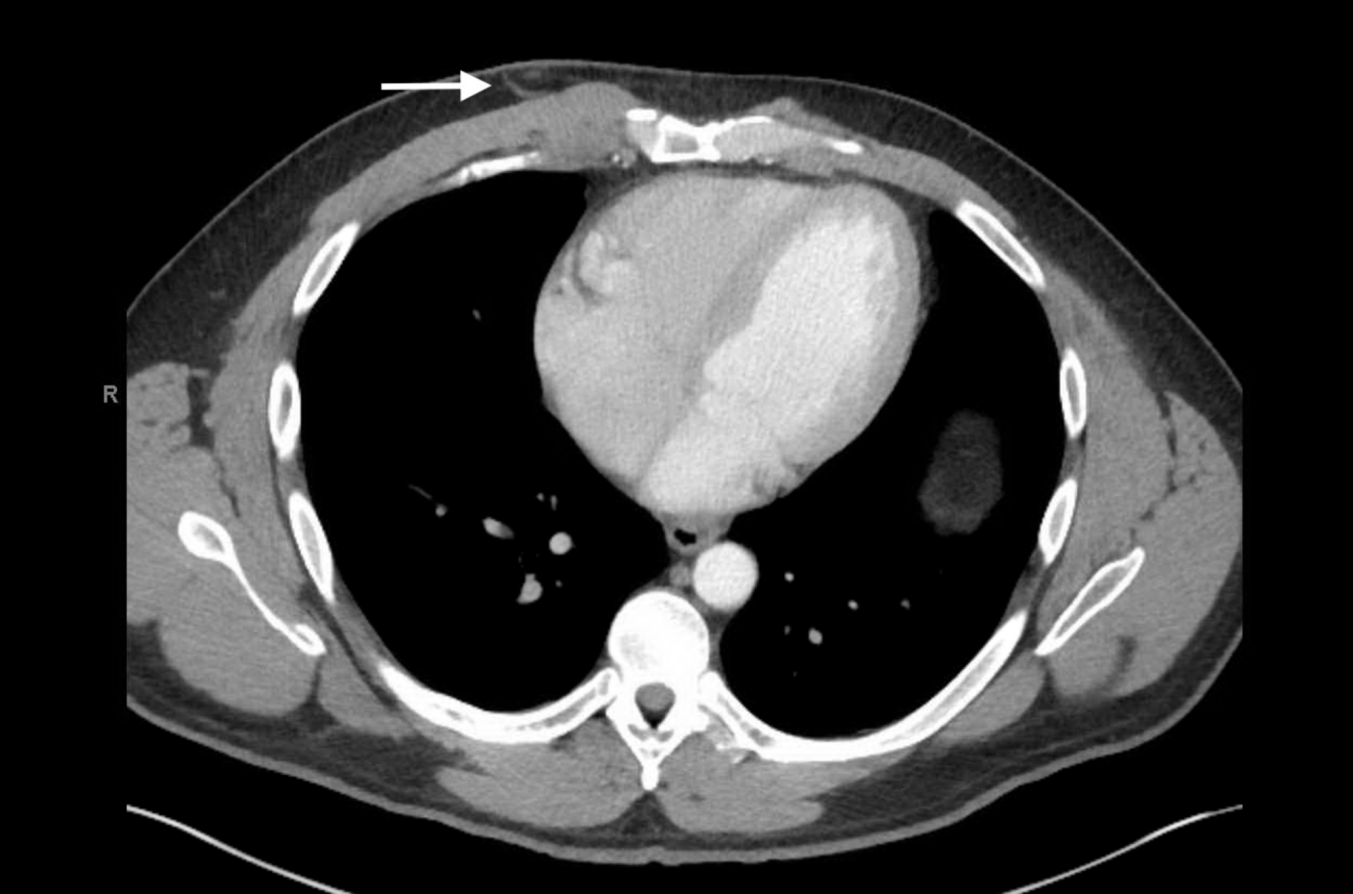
Computed tomography scan showing a subcutaneous thrombosis in the right chest wall.
